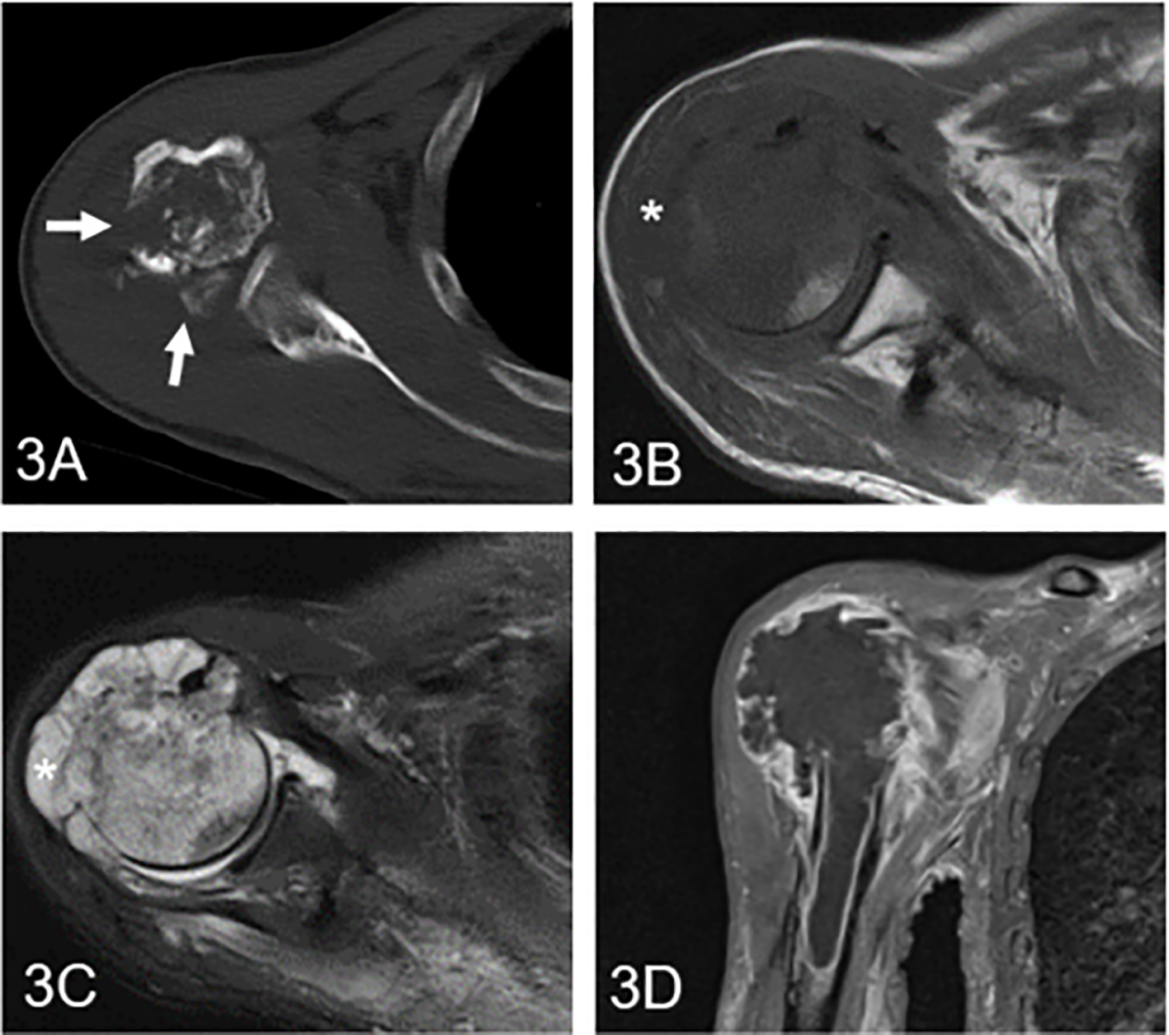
High-grade chondrosarcoma in the proximal humerus in a 61-year-old man. (A) Axial CT shows an ill-defined osteolytic lesion with multiple cortical destruction (arrow). (B) Axial T1WI presents an irregular soft tissue mass with an aggressive growth pattern (asterisk). (C) Axial fat-suppressed T2WI demonstrates a heterogeneous hyperintense mass with low signal components and soft tissue. (D) Coronal fat-suppressed contrast-enhanced T1WI shows diffuse and marginal enhancement.
